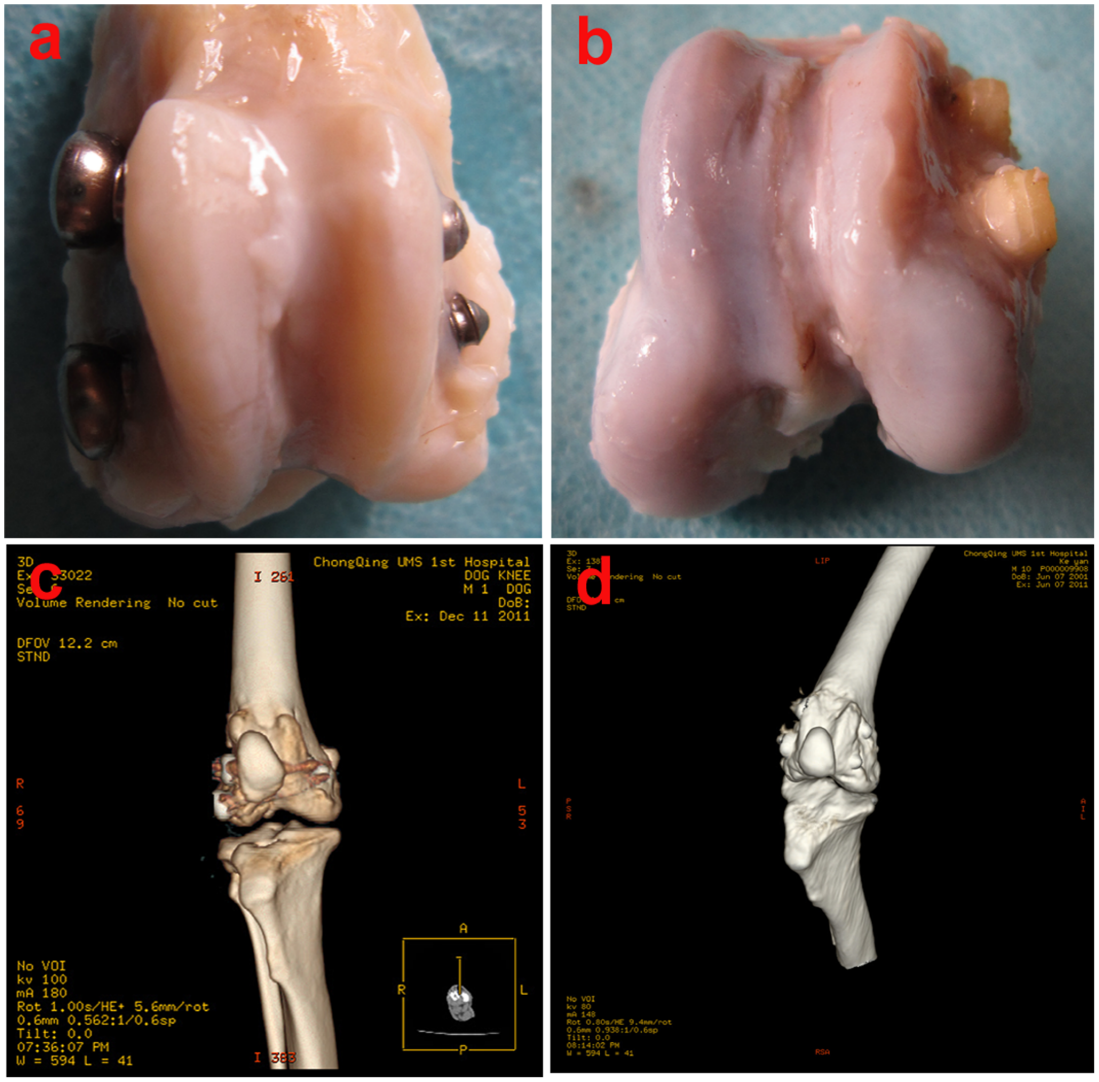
General photography and 3D-CT reconstruction images taken 12 weeks after operation. (a) General photography of metallic screws. (b) General photography of bioactive screws. (c) 3D-CT reconstruction image of metallic screws. (d) 3D-CT reconstruction image of bioactive screws.
